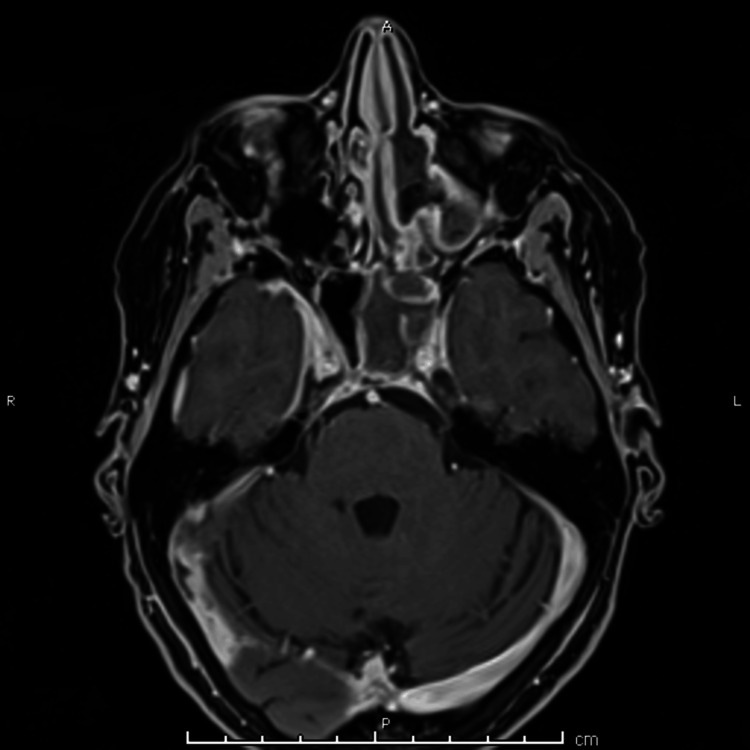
Axial view of MRI orbits with contrast showing left-sided ethmoid and sphenoid sinus opacification, consistent with findings from previous CT imaging
